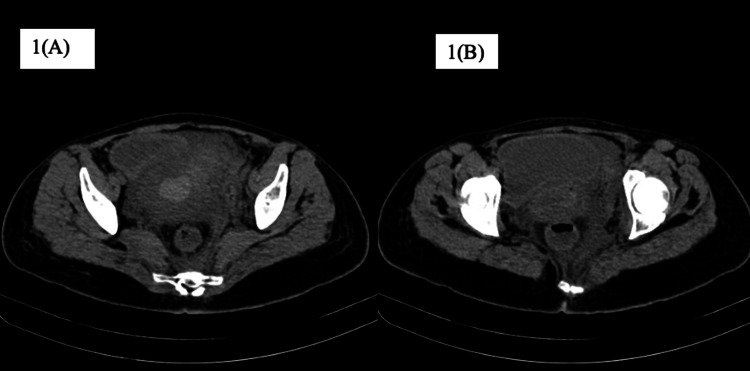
A: Contrast-enhanced CT showing hemoperitoneum on both sides of the uterus. B: Contrast-enhanced CT showing air pockets inside the uterus. CT: computerized tomography
